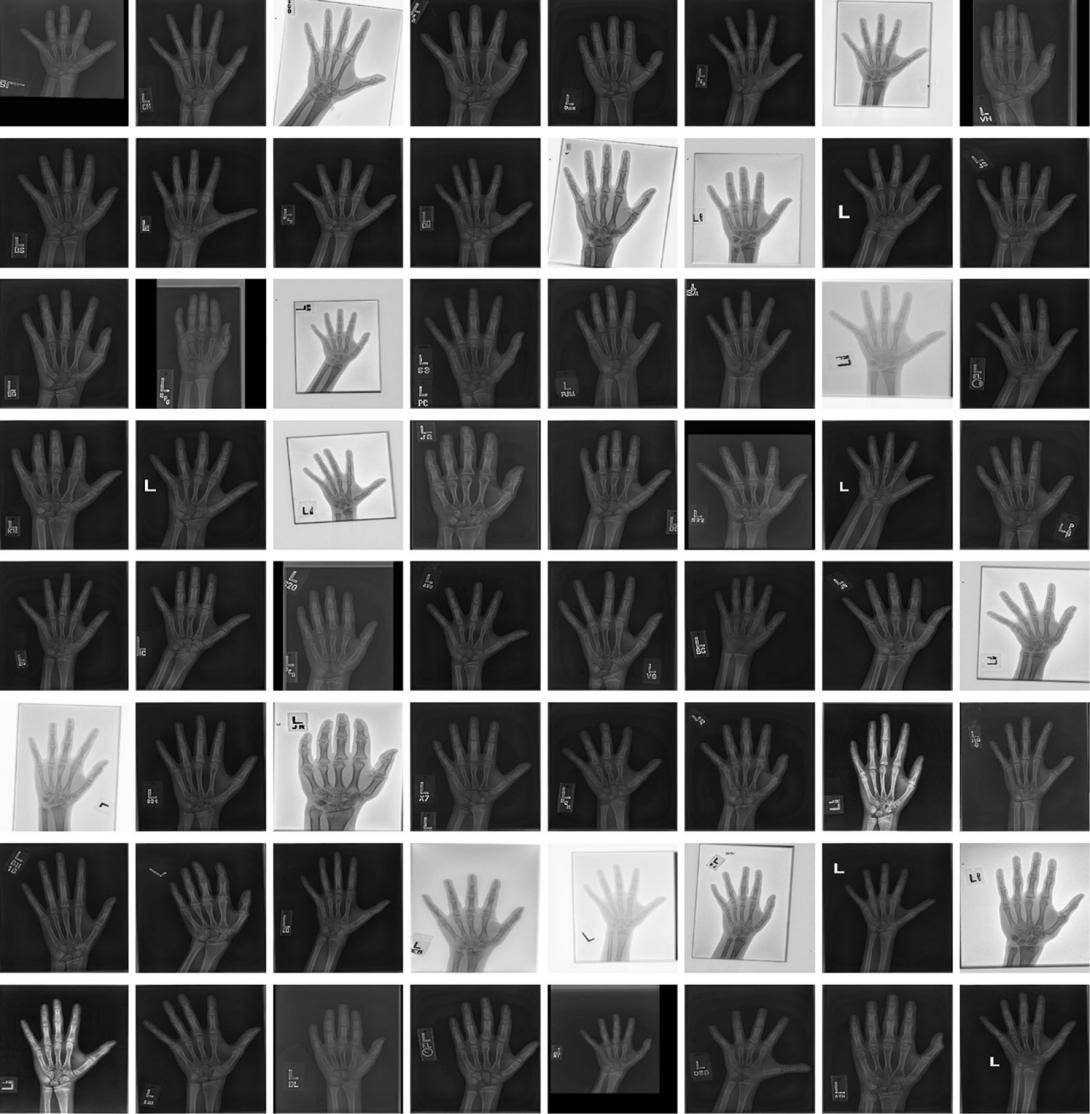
Examples of input radiographs utilized in this work. All images have varying sizes, but they were resized for the purposes of this figure
